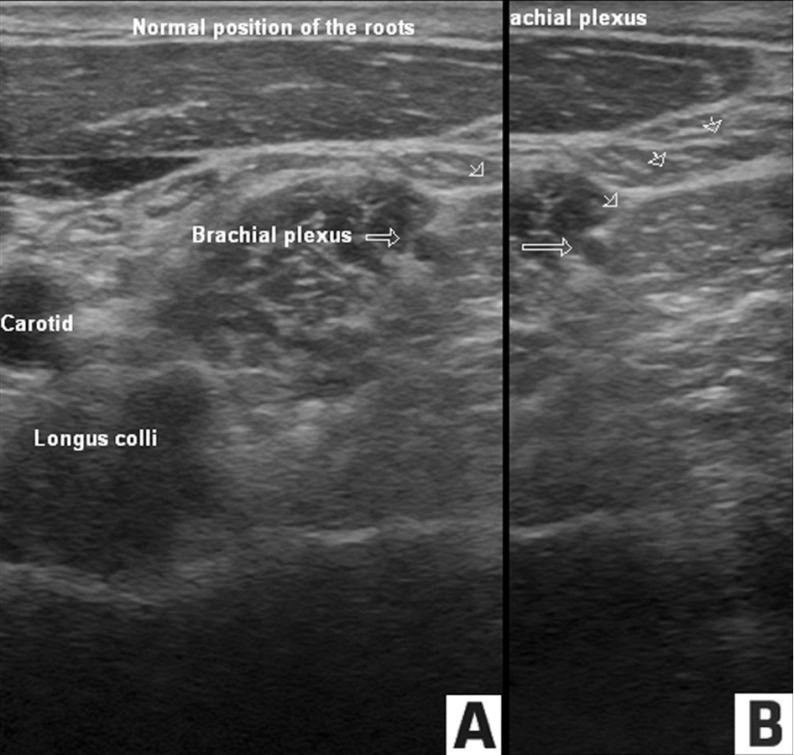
Ultrasound images over the anterior neck at the level of the brachial plexus roots. A) Normal position of the brachial plexus roots when a 25G needle (arrowheads) was just introduced above the brachial plexus roots (arrow). B) The fascial sheath enclosing the brachial plexus roots (arrow) is indented producing a lengthening of the distance, compared to figure 1A, when the needle (arrowheads) was advanced further. This also resulted in paresthesia.
